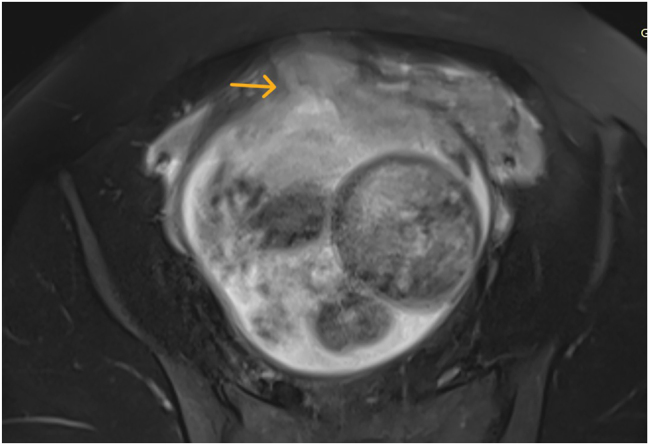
T1-weighted axial MRI showing hemorrhagic changes at the uterine rupture site.
